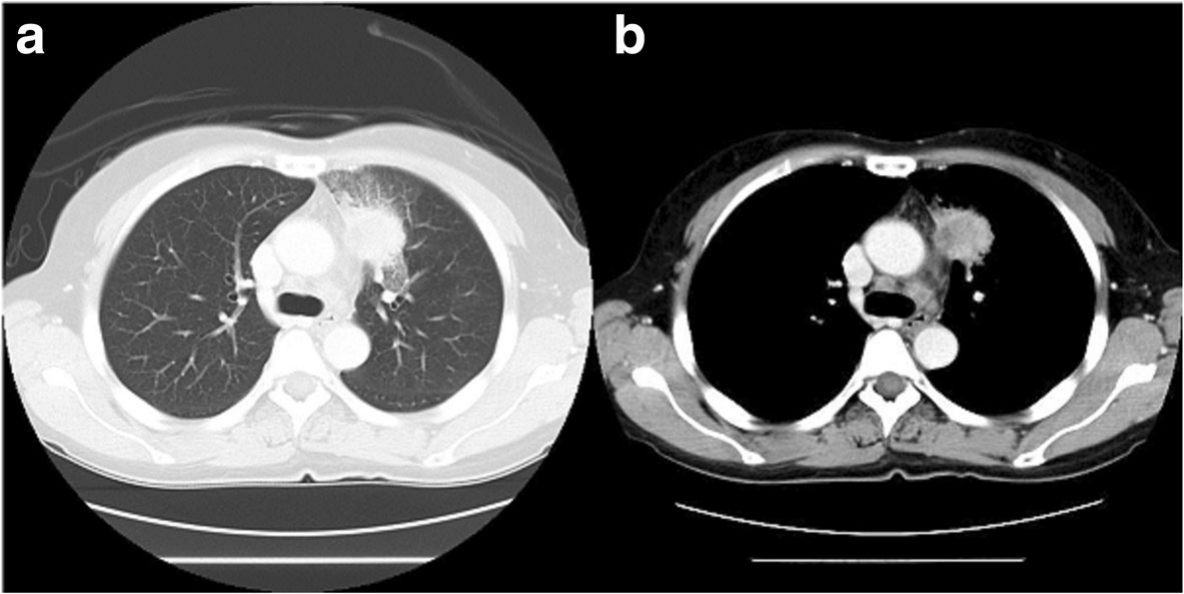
Preoperative computed tomography scan showing a 35 × 28-mm tumour in the anterior segment (segment 3) of the left upper lobe (a) and the anterior mediastinal fat tissue invaded by the tumour (b)
